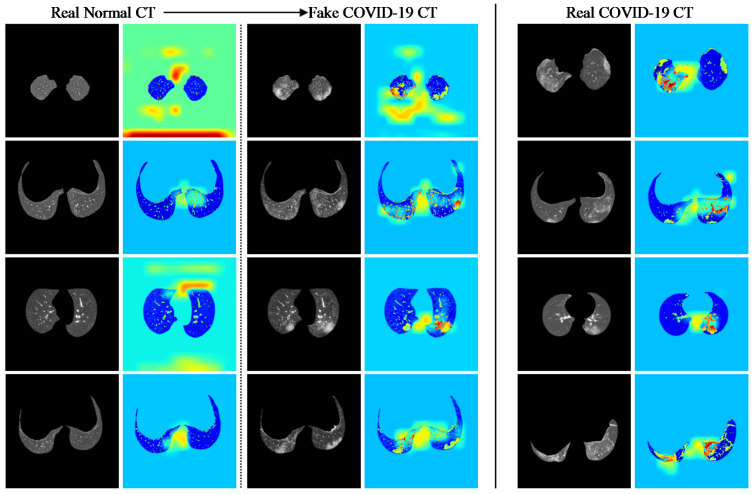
Grad-CAM of a pre-trained COVID-19 detection model on real non-COVID-19, real COVID-19, and synthetic COVID-19 CT images.
